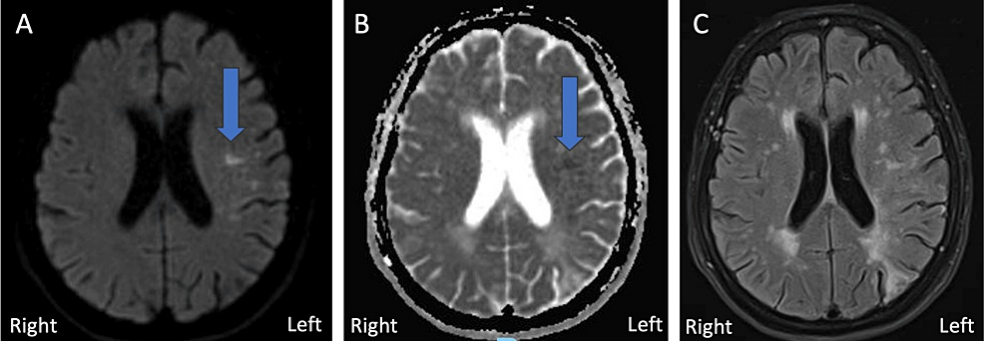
MRI A) Axial diffusion-weighted imaging. B) Axial apparent diffusion coefficient imaging. C) Axial T2 fluid-attenuated inversion recovery imaging. Blue arrows show the location of restriction diffusion with apparent diffusion coefficient correlate MRI: magnetic resonance imaging
